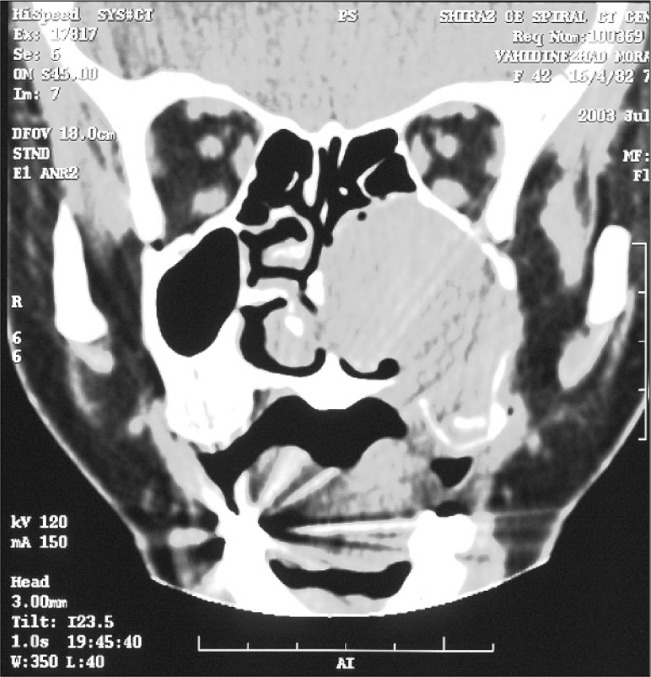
Coronal computed tomography scan from the nose and paranasal sinuses, showing a large mass in the left maxillary sinus with extension to the nasal cavity, in a 41-year-old woman.
